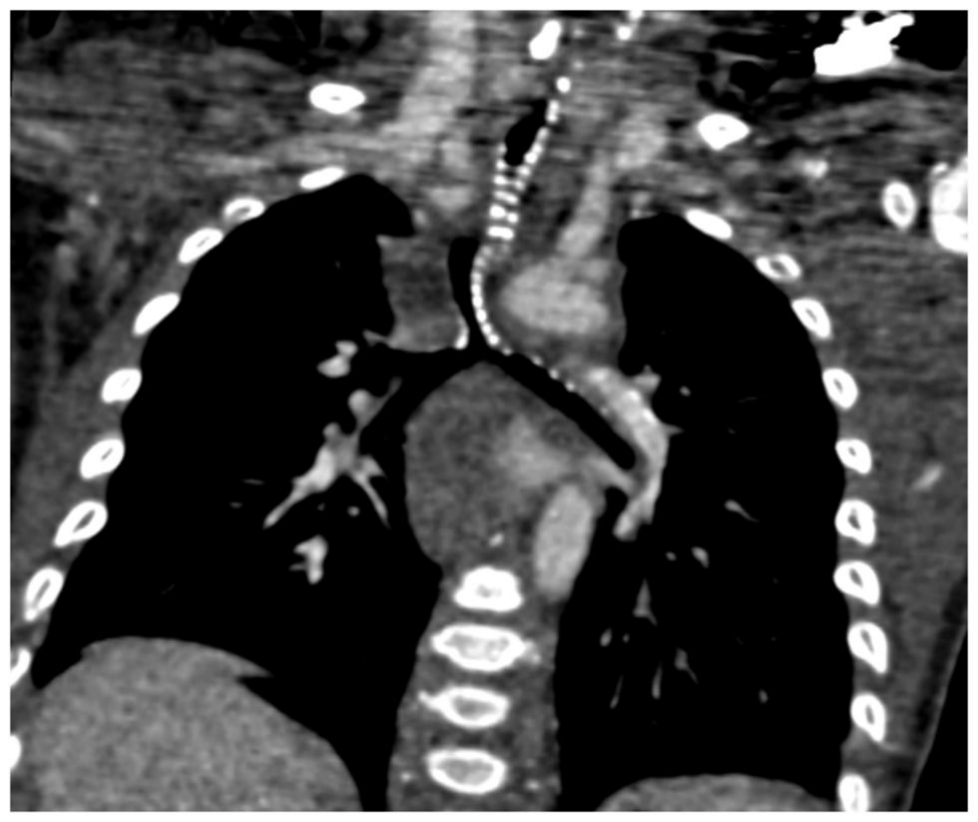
A 7-month old infant with pulmonary hypertension was demonstrated tracheobronchial widespread stenoses at CT and the fiberscope could not pass the lesion. CT coronal image demonstrated the widespread ossification of tracheobronchial cartilage and irregular stenoses.
